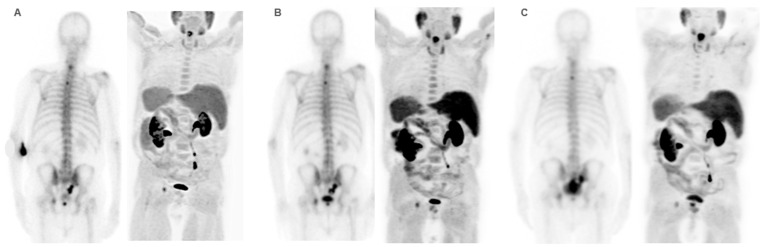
Patient ≠ 2. An 88-year-old man diagnosed with metastatic prostate cancer, Gleason Score 7 (4 + 3), from the onset. He received first-line treatment with Enzalutamide until biochemical and bone progression after 5 years. Treatment with ^223^Ra was proposed as second-line. Baseline BS and FCH PET/CT (A) show oligometastatic disease with only axial involvement and predominantly osteoblastic. At the start of ^223^Ra treatment, the patient was in very good general condition (ECOG 0) with baseline PSA levels of 14.9 ng/mL, AP of 73 IU/L, and LDH of 450 IU/L. The patient completed 6 doses of ^223^Ra, remaining stable in the interim BS and FCH PET/CT studies (B) but showed bone progression at the end of treatment in both the BS and FCH PET/CT (C). His PSA levels increased during the treatment, while AP levels remained stable. After ^223^Ra treatment, the patient received Docetaxel and experienced a bone event that consisted of a painful metastatic bone at 17 months, treated with palliative vertebral radiotherapy. He ultimately died with an OS of 30 months.
